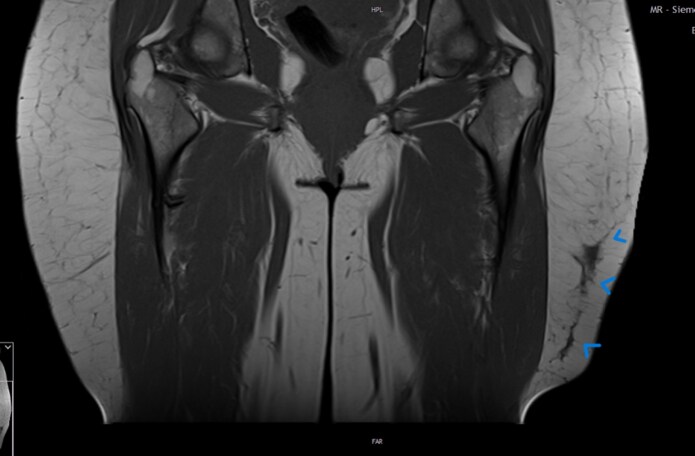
MRI image of the thighs performed at the time of initial presentation, ∼2 years postinjury. Arrows mark the subcutaneous cleft that represents the Morel-Lavallée lesion.
